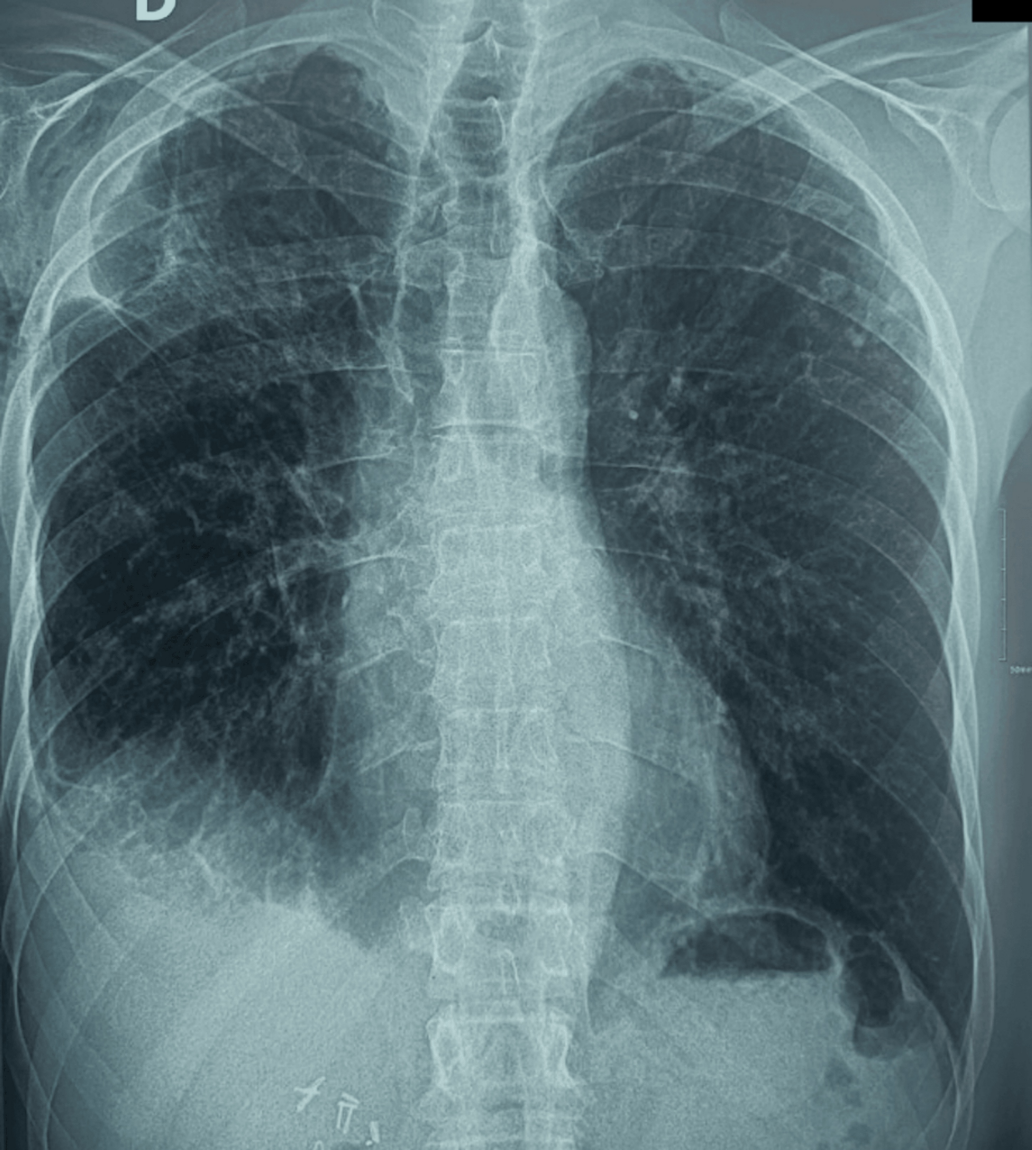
Postoperative chest X-ray of our patient Postoperative chest X-ray showing normal findings in our patient after surgery.
